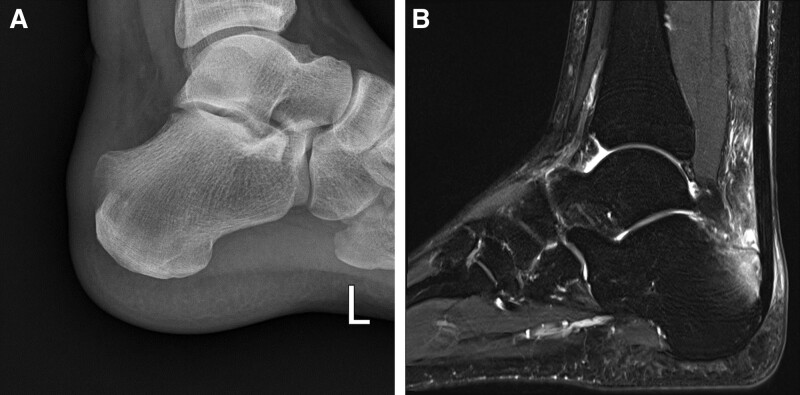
A 27-year-old male with insertional Achilles tendinopathy. The preoperative ankle lateral plain radiogram shows Haglund’s deformity. MRI shows the retrocalcaneal bursa and mid-substance, the insertion area of the Achilles tendon, and thickening of the Achilles tendon. MRI = magnetic resonance imaging.
